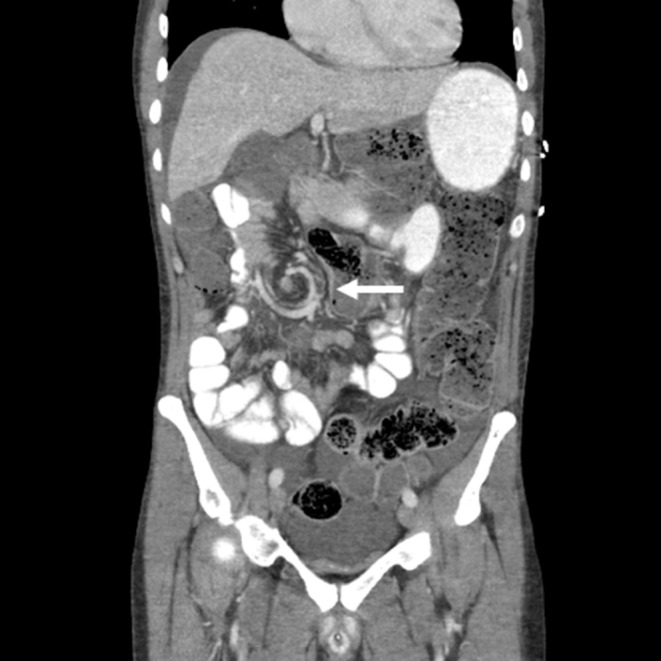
A CT scan of the abdomen demonstrates a “whirl sign” which is when the bowel rotates arounds its mesentery leading to whirls of the mesenteric vessels.
